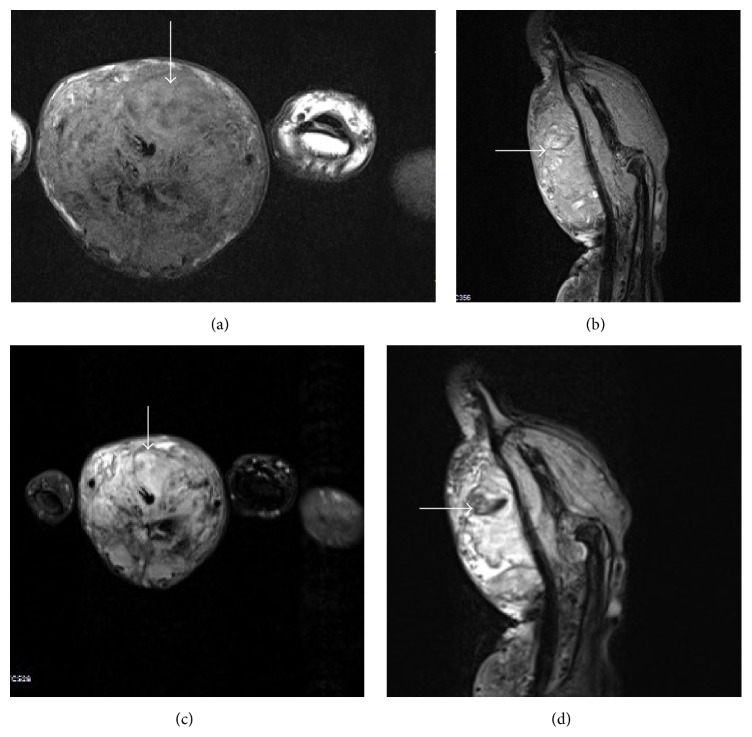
AVM. MRI (of the same patient as in Figure 1) shows a large heterogeneous T1W hypointense (a) and T2WFS hyperintense (b) lesion (arrows) showing few intralesional flow voids, marrow extension, and cortical breech. On post contrast T1WFS sequences ((c), (d)) the lesion shows intense heterogeneous postcontrast enhancement (arrows).
